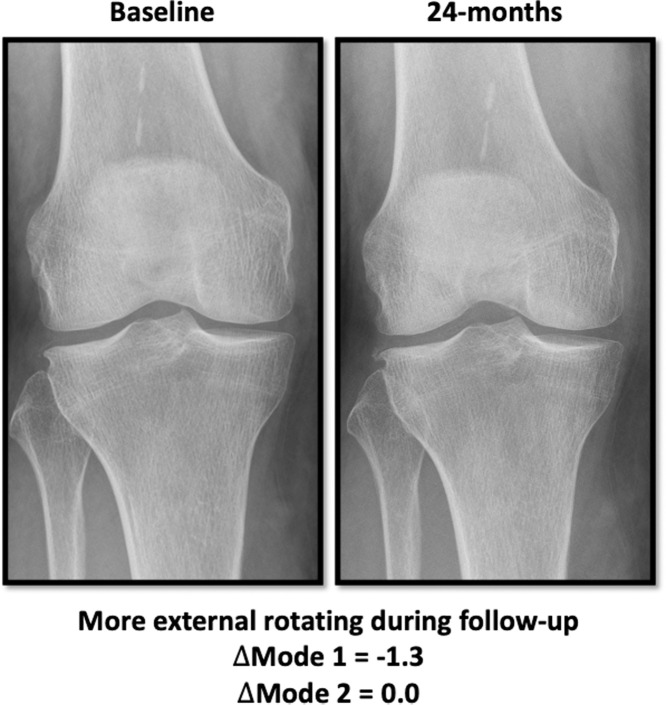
Example of knee radiographs of the same patient at baseline (left) and 24-month follow-up (right). At follow-up, the knee is positioned in greater external rotation, as indicated by a change in shape mode 1 (ΔMode 1 = –1.3), while the degree of flexion/extension remains unchanged (ΔMode 2 = 0.0). SSMs allow for the quantification of such subtle differences in knee positioning, which are often not visually apparent.
